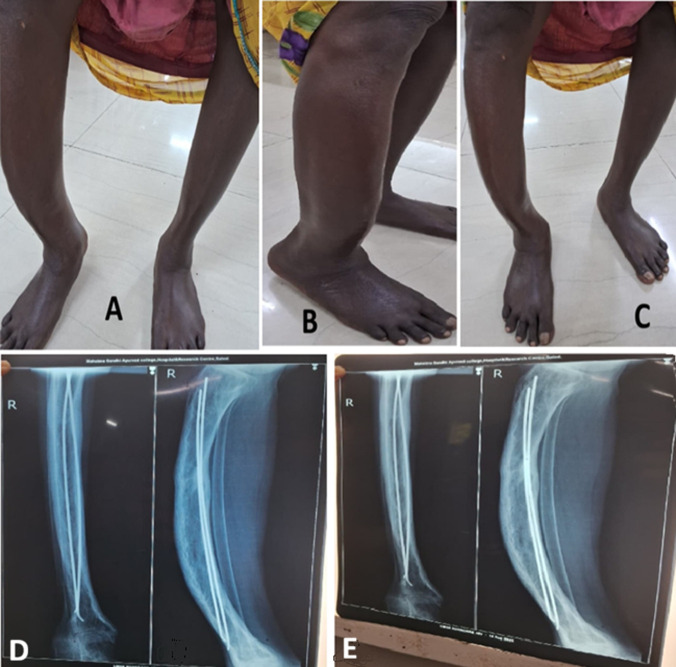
A) shape of the right leg from the medial aspect; B) shape of the right leg from the lateral aspect; C) shape of the right leg from the anterior aspect; D, E) radiograph X-ray of the right leg with an implant
